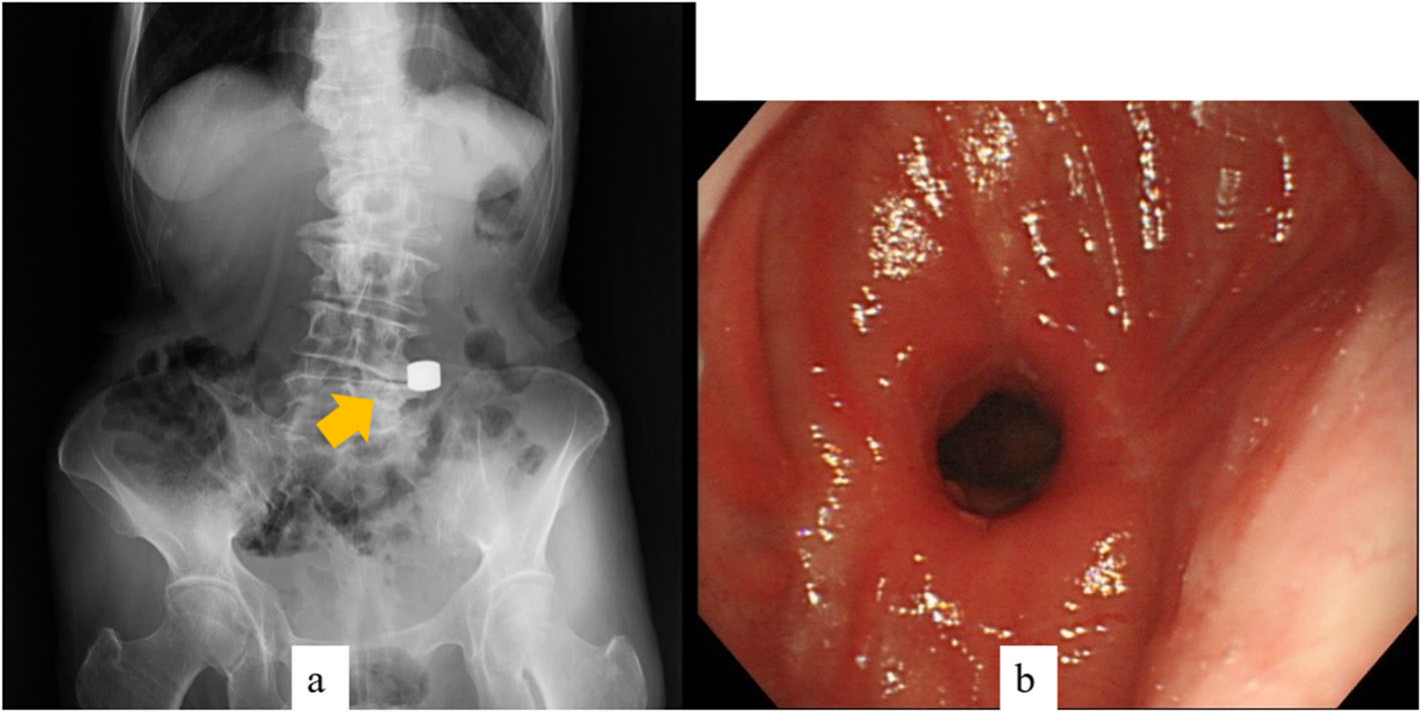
Imaging of the created anastomosis. a Plain X-ray imaging: two magnets have moved to anal side (arrow). b Anastomosis observed by esophagogastroduodenoscopy after removal of the magnets
